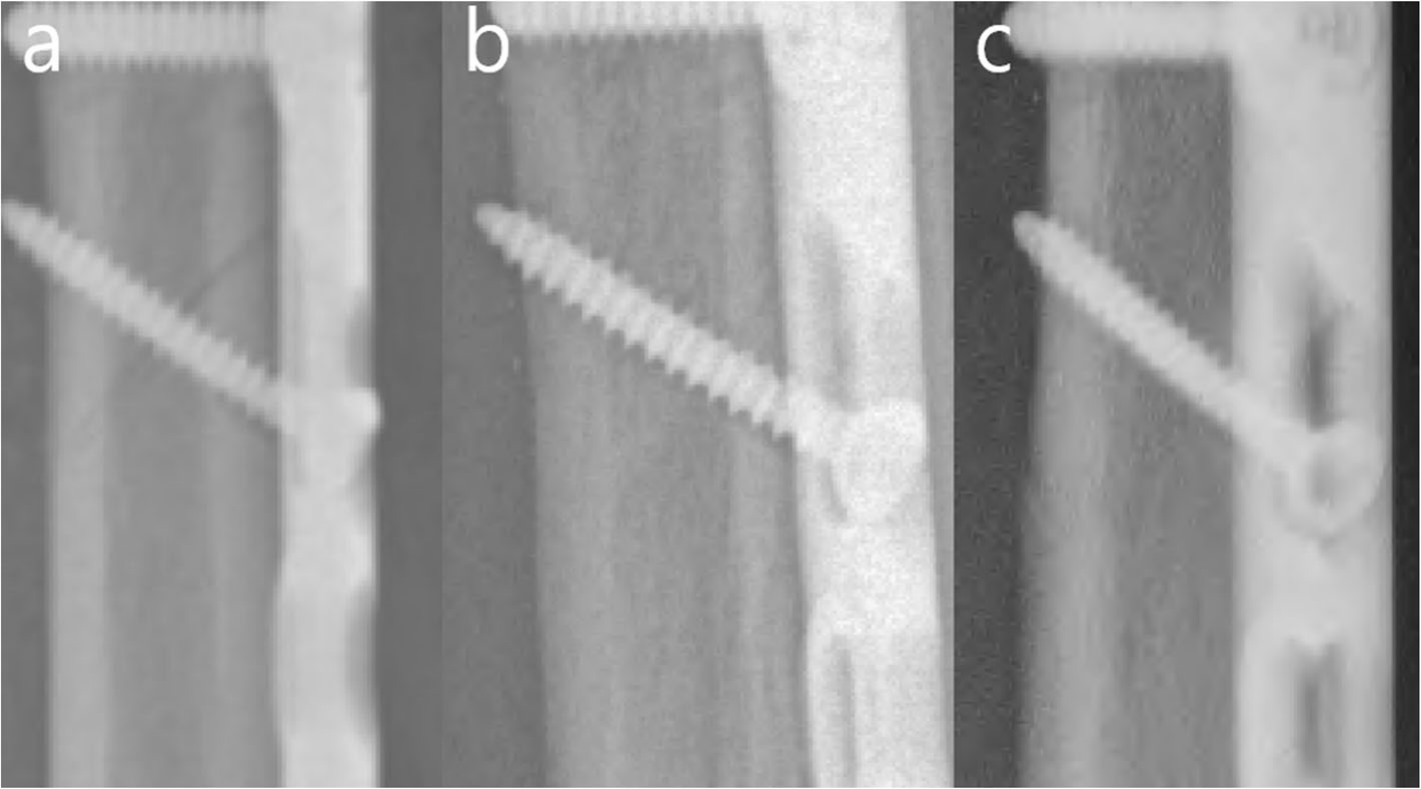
Serial radiographs of a guided osteotomy with paralleled osteotomy and minimal osteotomy reduction gap. a Immediate postoperative radiograph demonstrating that the lag screw could enhance the compression and stability of the osteotomy. b After 2 months, the osteotomy is united. c At 10 months, complete osteotomy consolidation is achieved.
